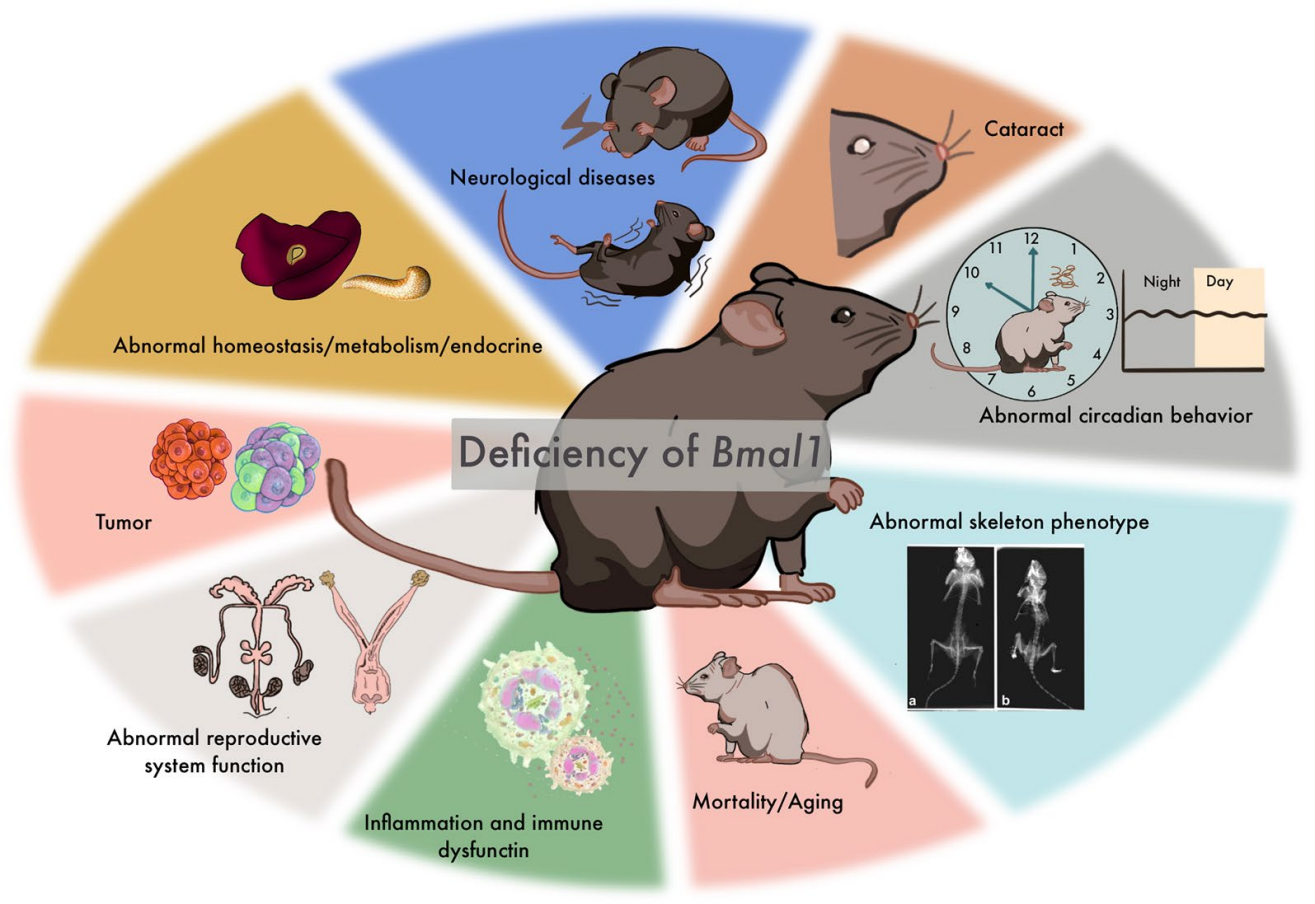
A brief overview of the basic functions of *Bmal1. Bmal1* has important roles in circadian rhythm, vision/image processing, the neurological basis of behaviors, endocrine/exocrine glands, liver/biliary system, homeostasis/metabolism, oncogenesis and tumorprogression, respiratory system, immune system, growth and aging, skeletal system development. Radiographs are from [18]
